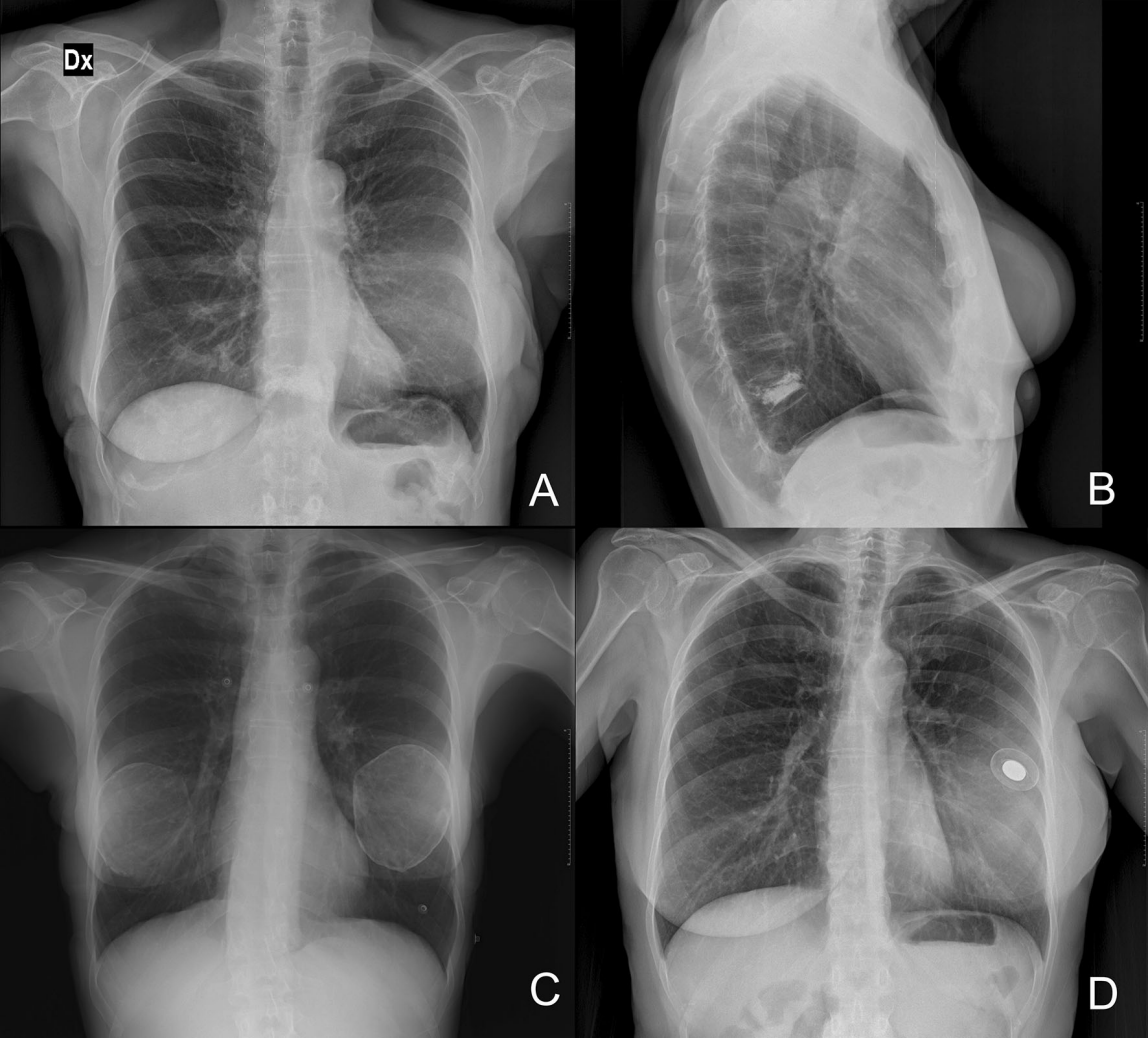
The de Boer et al. criteria for identifying breast implants on chest X-rays. They include projection lines that follow the contour of the implant within the breast, as seen on a posteroanterior (A) and latero-lateral view (B); calcifications along the periprosthetic capsule (C); and the metal magnetized valve/port of a tissue expander (D)
